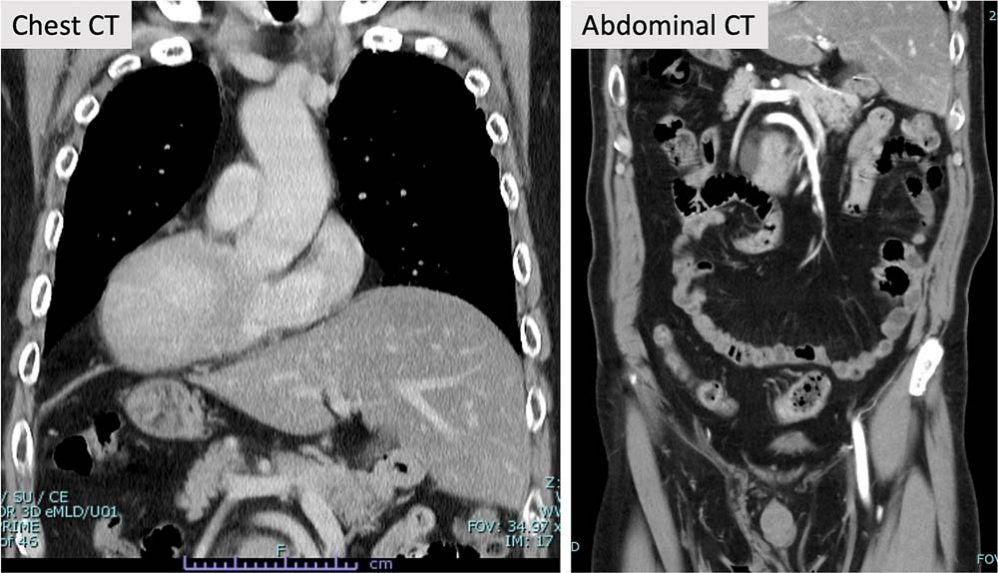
Contrast-enhanced CT. Contrast-enhanced CT revealed SIT. Other abnormalities in the blood vessels were not evident in the chest and abdomen.
